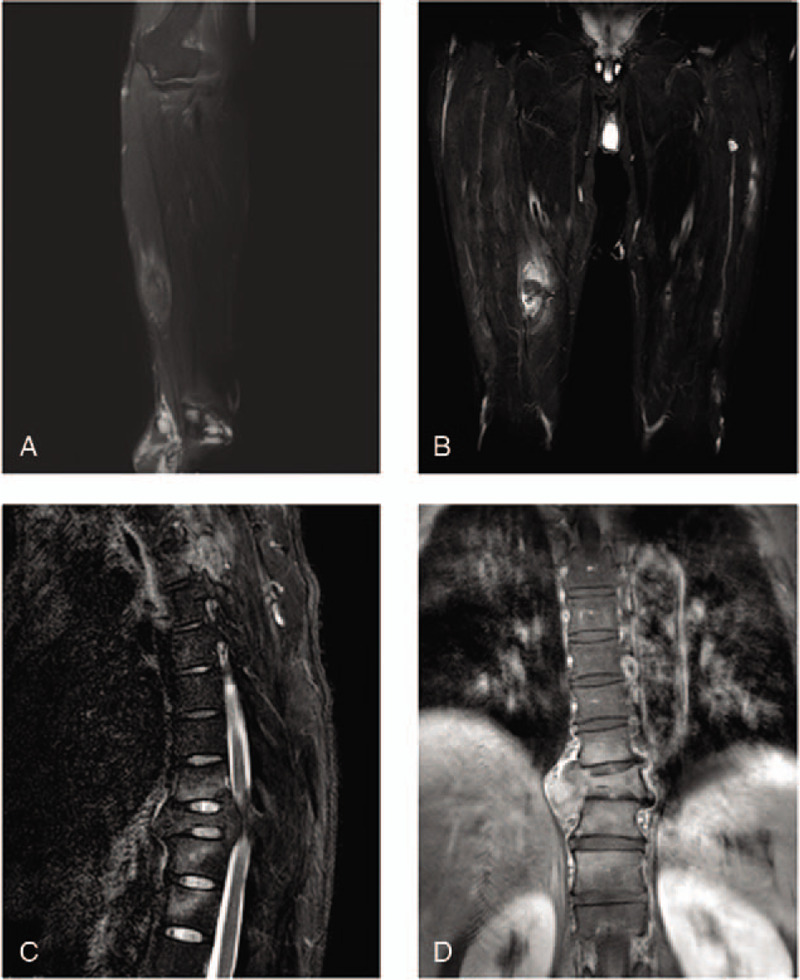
Magnetic resonance images. (A) A lump can be seen on the ulnar side of the left forearm muscle with a slightly higher signal in the T2-fat-suppression images. (B) A lump can be seen in the middle part of the right thigh with an equal signal in fat-suppression images, and a high signal in the peripheral muscle tissue. (C) The body of the 9th thoracic vertebrae is flattened and wedge shaped. There is a high patchy fat-suppression signal in the spinal cord. (D) A strip-like abnormal signal and mild uneven enhancement in the soft tissue around the 9th thoracic vertebrae can be observed in an enhanced scan.
